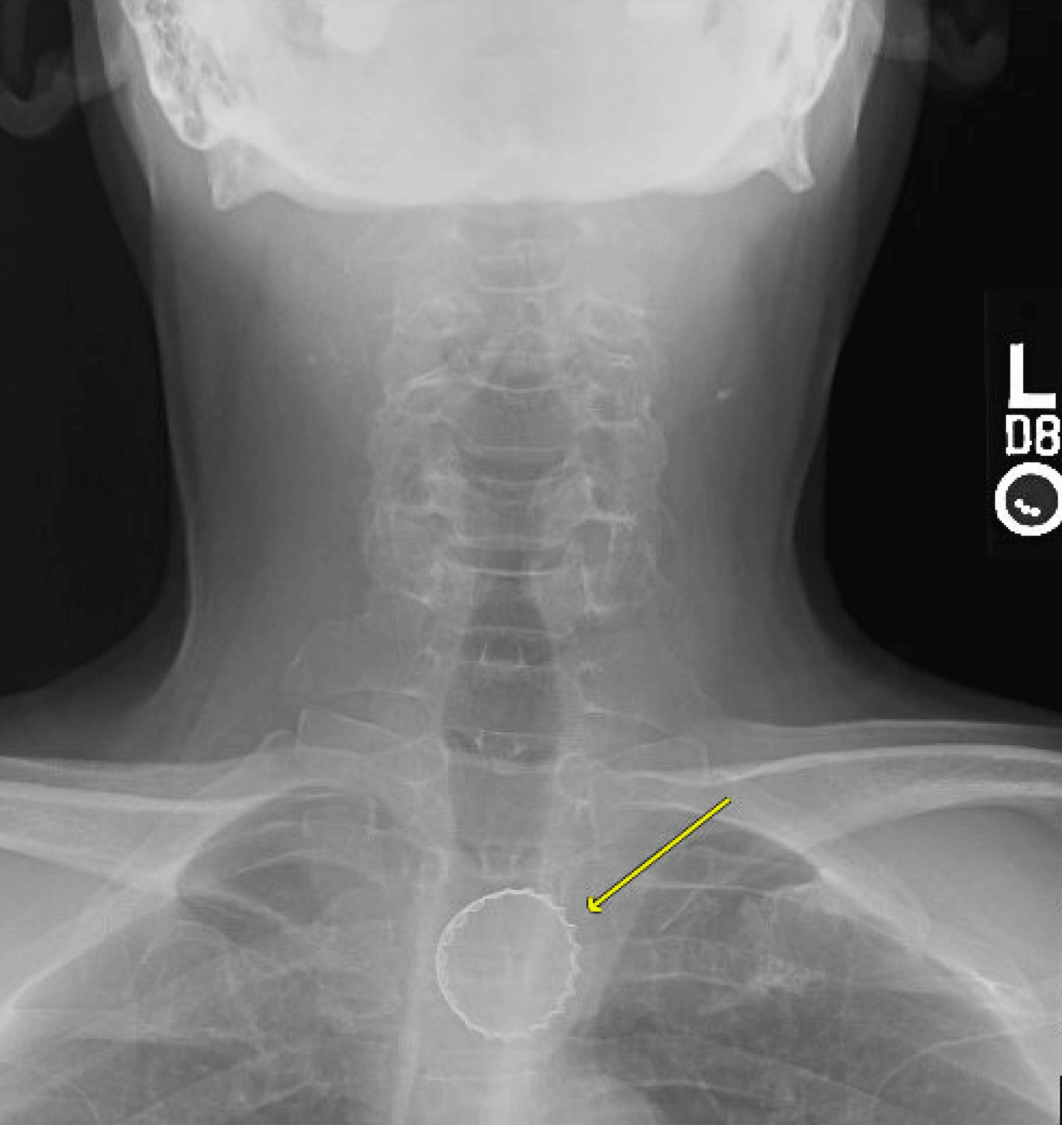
An X-ray of the cervical spine (anteroposterior (AP) view) A radiopaque circular body projecting over the upper esophagus was noted.
